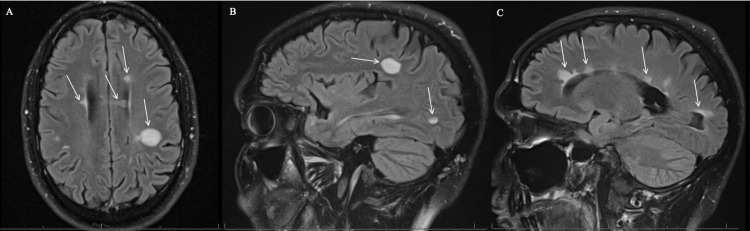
(A-C): MRI brain with and without contrast. Figure 2A showed significantly decreased vasogenic edema associated with the previously described left parietal lobe white matter lesion. Additional supratentorial white matter lesions consistent with MS are again appreciated and not significantly changed. Figures 2B and 2C showed two sagittal views of the decreased vasogenic edema associated with the previously described left parietal lobe white matter lesion. Additional supratentorial and infratentorial white matter lesions consistent with MS are again appreciated and not significantly changed.
